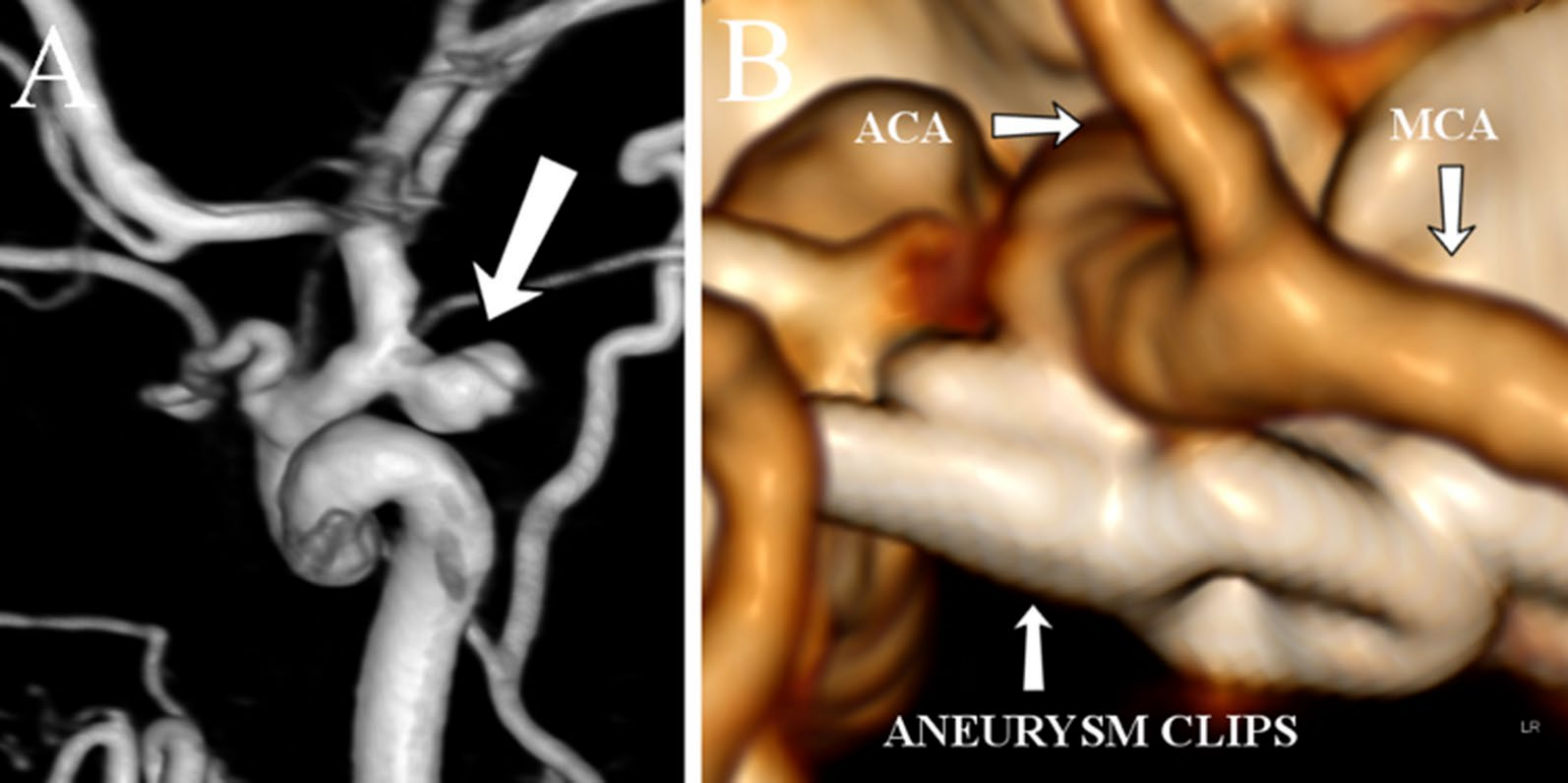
A conventional catheter angiography showed a right posterior communicating artery aneurysm (a; white arrow). After surgery, computed tomography angiography demonstrated that the aneurysm was clipped (b). MCA = middle cerebral artery, ACA = anterior cerebral artery (Color figure online)
